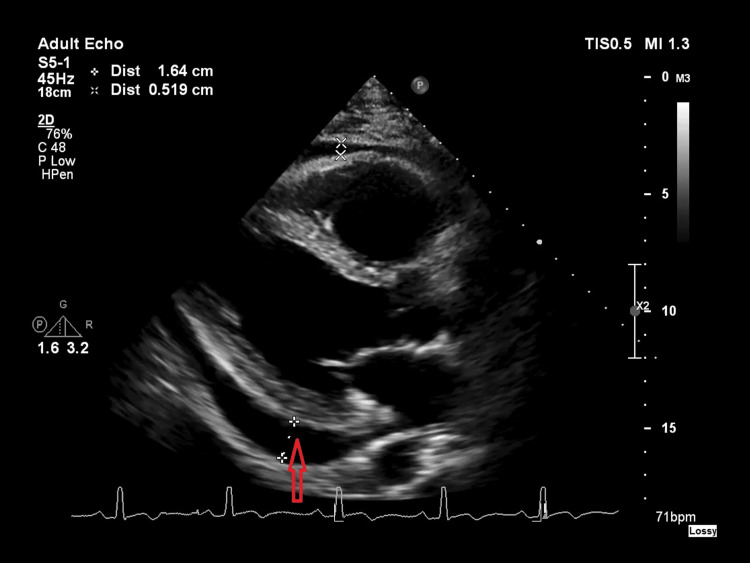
Echocardiography parasternal long axis view. The figure shows moderate circumferential pericardial effusion, with a maximum diameter of 1.6 cm posterior to the left ventricle.
